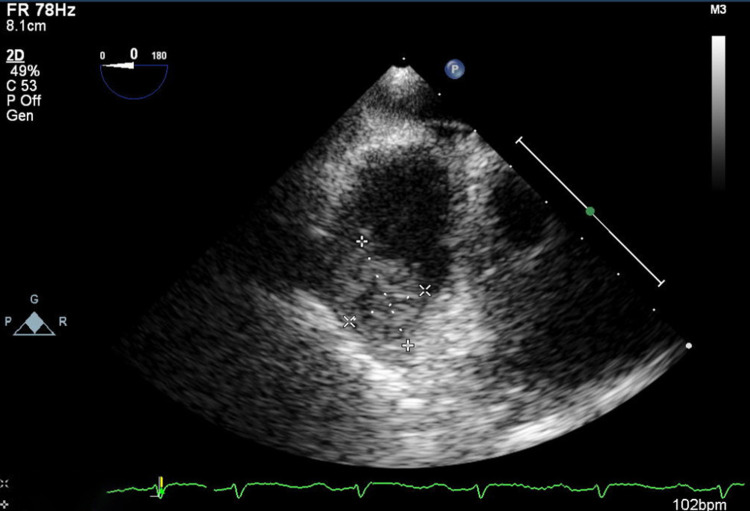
Transesophageal echocardiogram. Sessile heterogeneous irregular mass of 32 x 18 mm occupying the right atrium.
